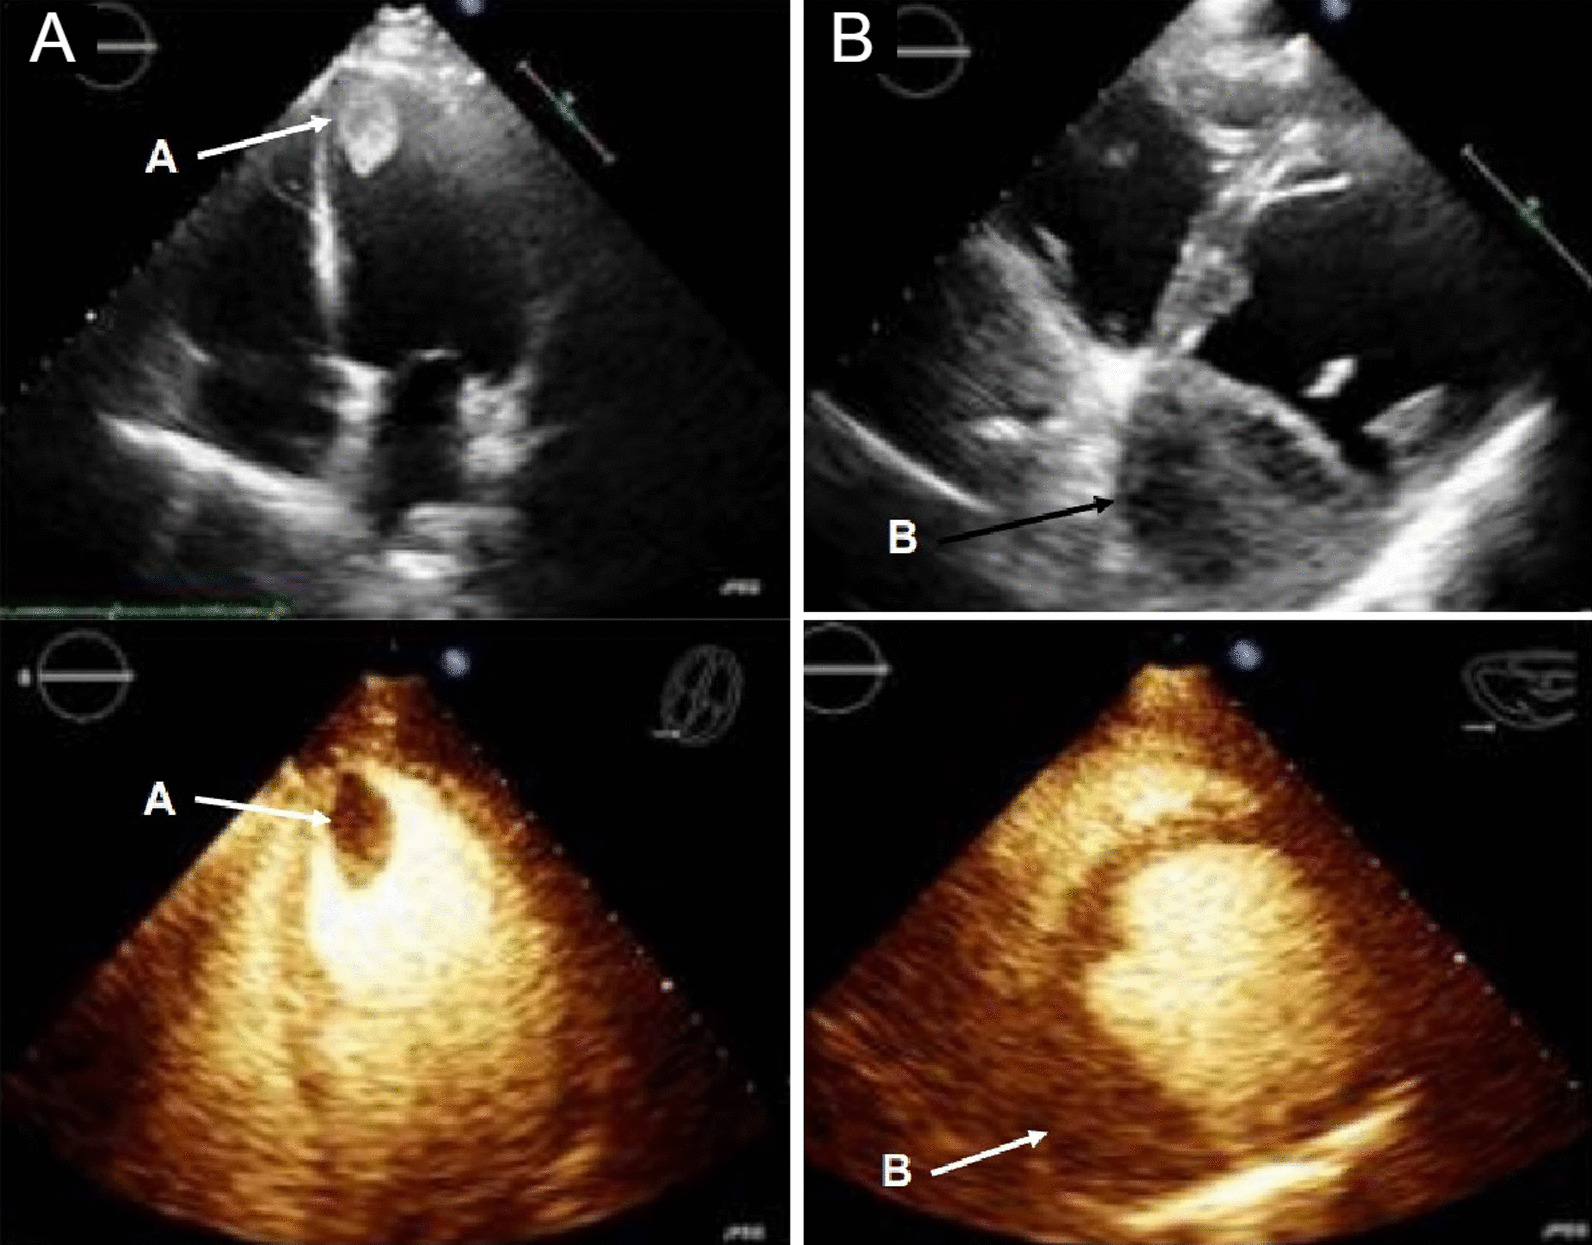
Case 8. Figure 4. Transthoracic echocardiogram (TTE) four chamber A and short axis B views. Non contrast and contrast enhanced views showed the presence of the masses in the LV apex A and inferior wall (B)
